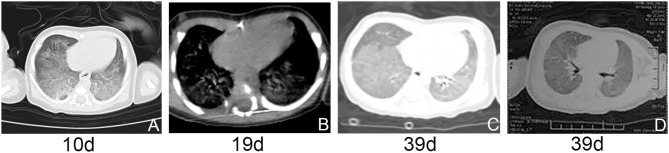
(A–D) HRCT and airway reconstruction showed decreased transmittance and ground glass opacity in bilateral lungs.
